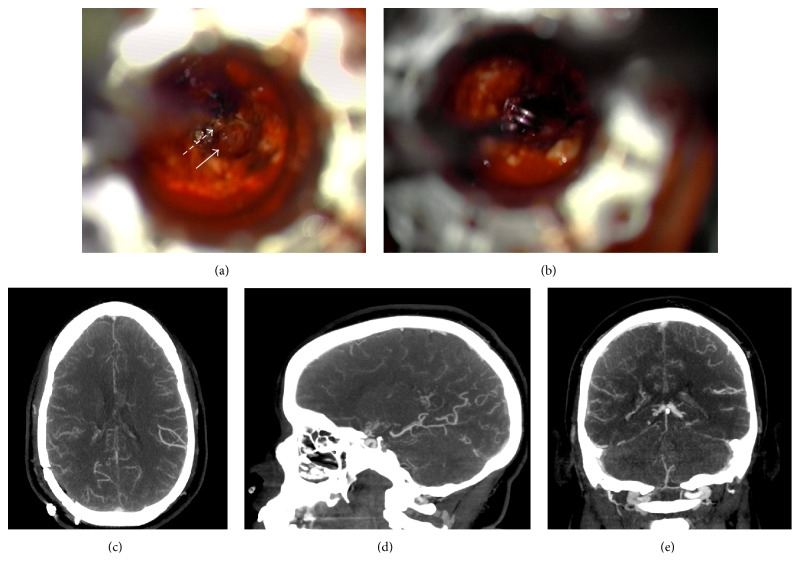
(a) Intraoperative view through the outer sheath of the BrainPath endoport system shows exposure of the proximal vessel (dashed arrow) and the partially thrombosed, fusiform periventricular aneurysm (solid arrow) within the atrium of the right lateral ventricle. (b) A temporary clip was placed on the inflow vessel to achieve proximal control prior to ligation of the aneurysm with electrocautery. Postoperative CTA, (c) axial, (d) sagittal, and (e) coronal views, shows interval decrease in the quantity of intraventricular hemorrhage and no evidence of residual aneurysm.
